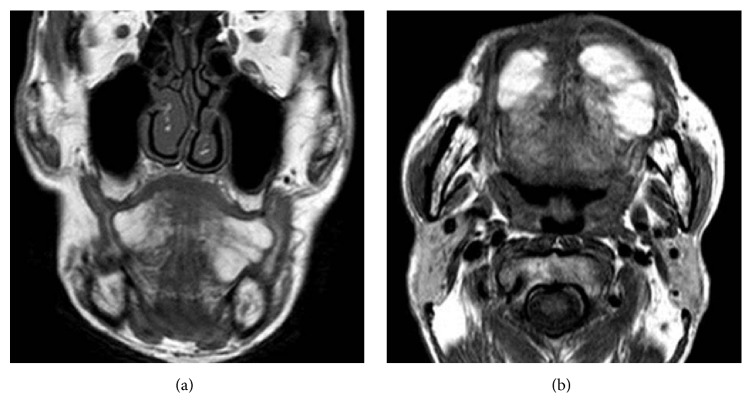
MR images ((a) axial view, (b) horizontal view). MR imaging shows high-intensity nodules and adipose tissue invading into the bilateral lingual muscle.
